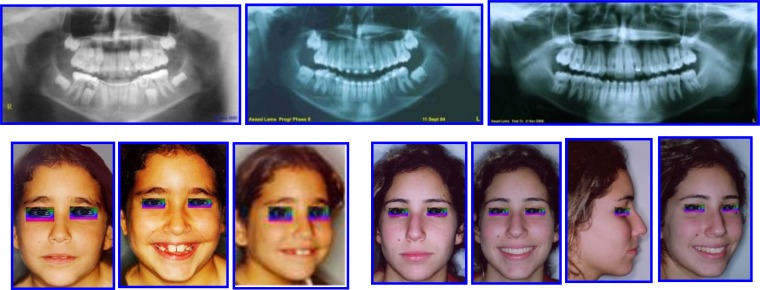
Pretreatment records: Facial & occlusal features. Radiographs: Ceph analysis & Panoramic. Case # 3.
